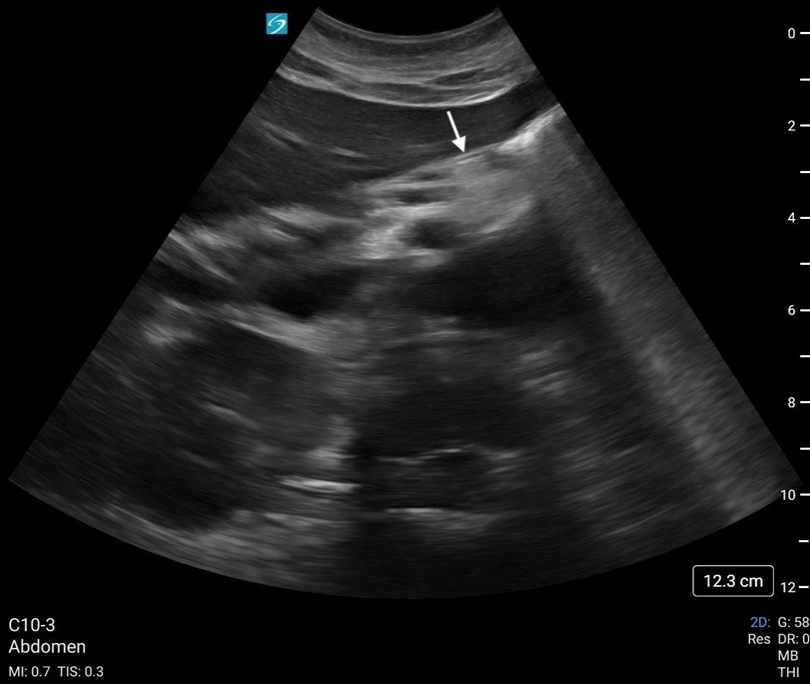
Enteral feeding tube (white arrow) visualized in the immediate post-pyloric region using point of care ultrasound (POCUS)
